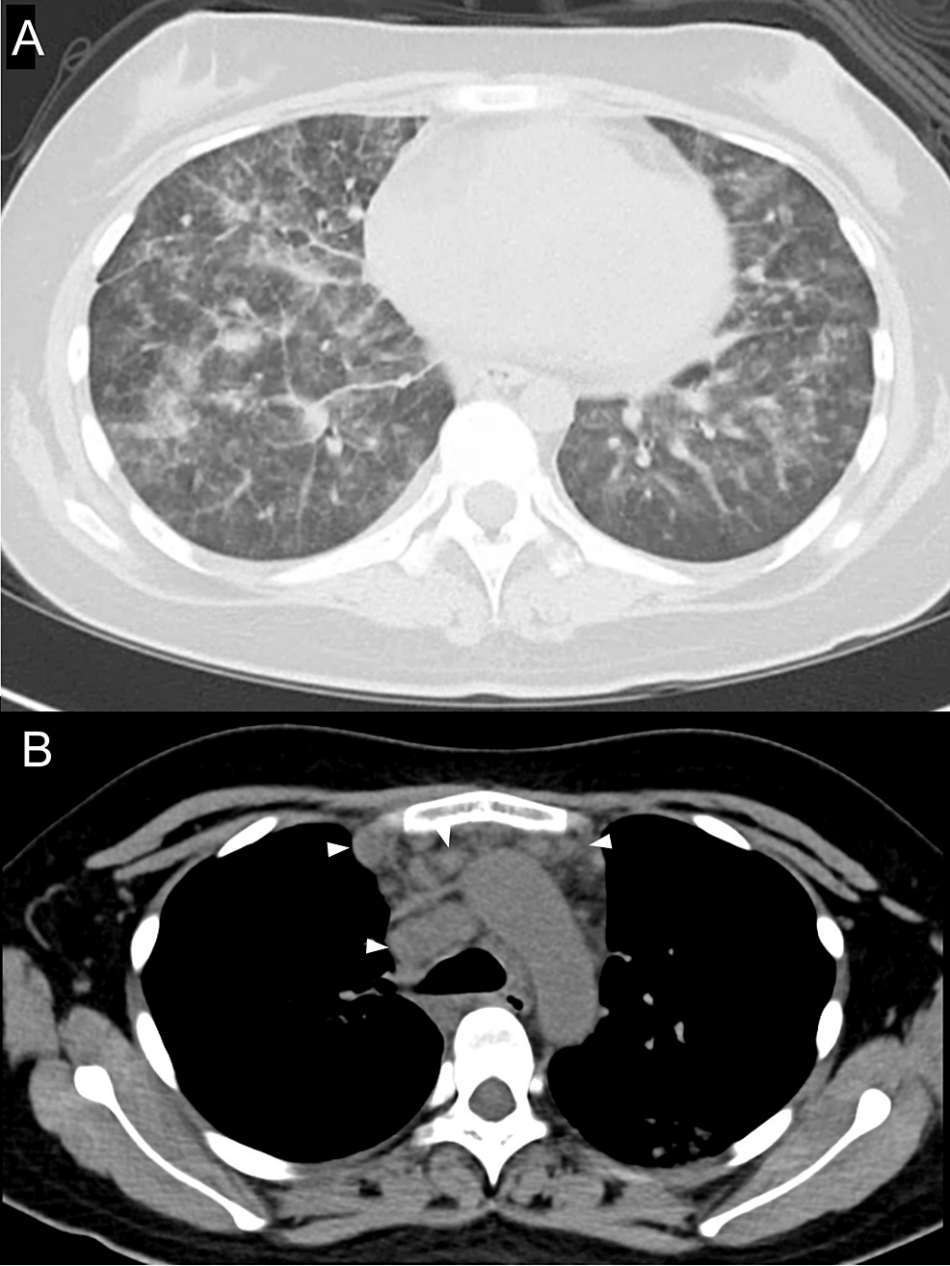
Computed tomography of the chest A. Computed tomography of the chest revealed diffuse infiltrations with focal consolidation. There were no nodular lesions or cavitation. B. The swelling of multiple mediastinal lymph nodes was conspicuous on mediastinal window views (arrows).
